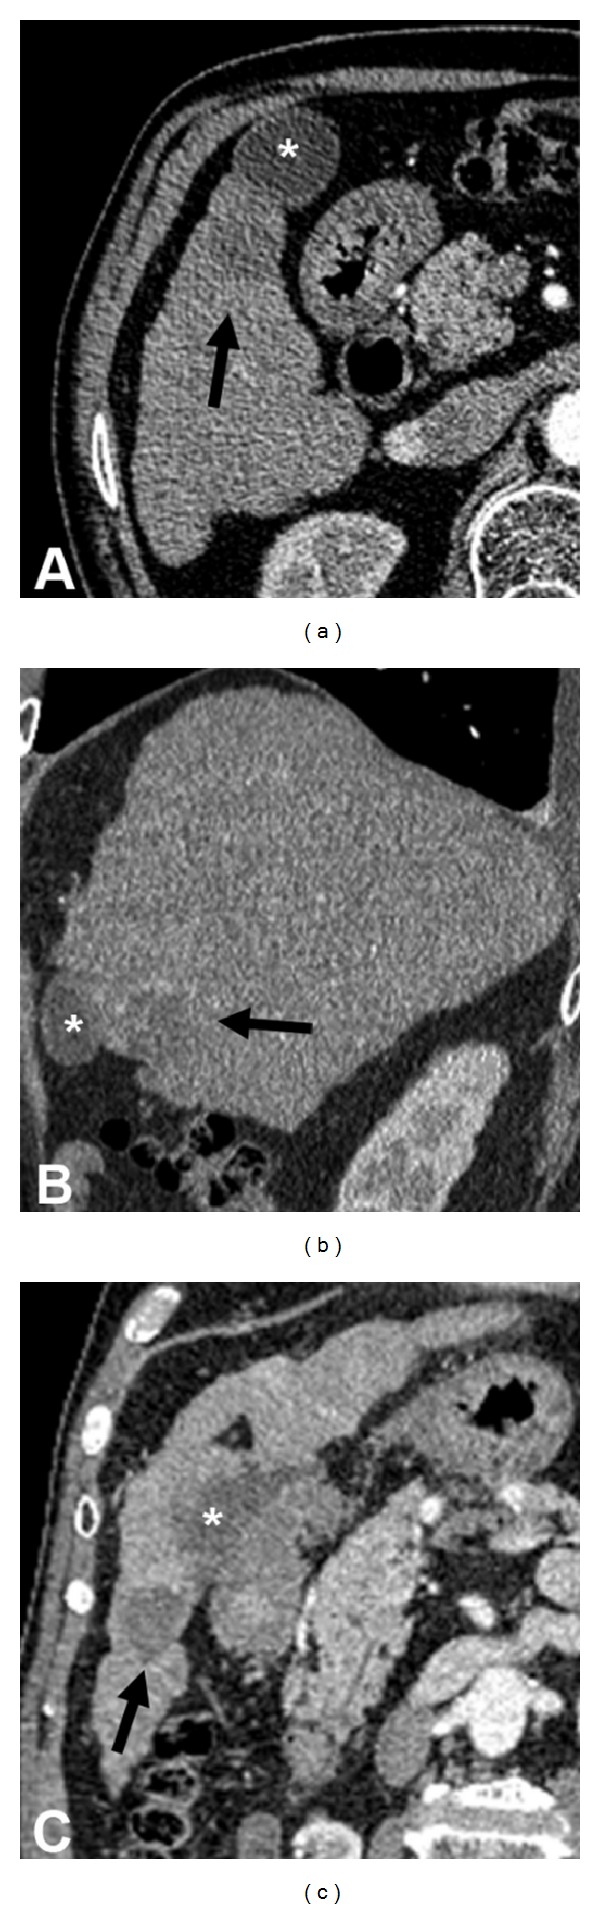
Axial CT image (a) and CT multiplanar reconstructions (b, c), performed after six months, show the absence of contrast medium enhancement of the ablated liver lesion (black arrow) without gallbladder (∗) damage.
